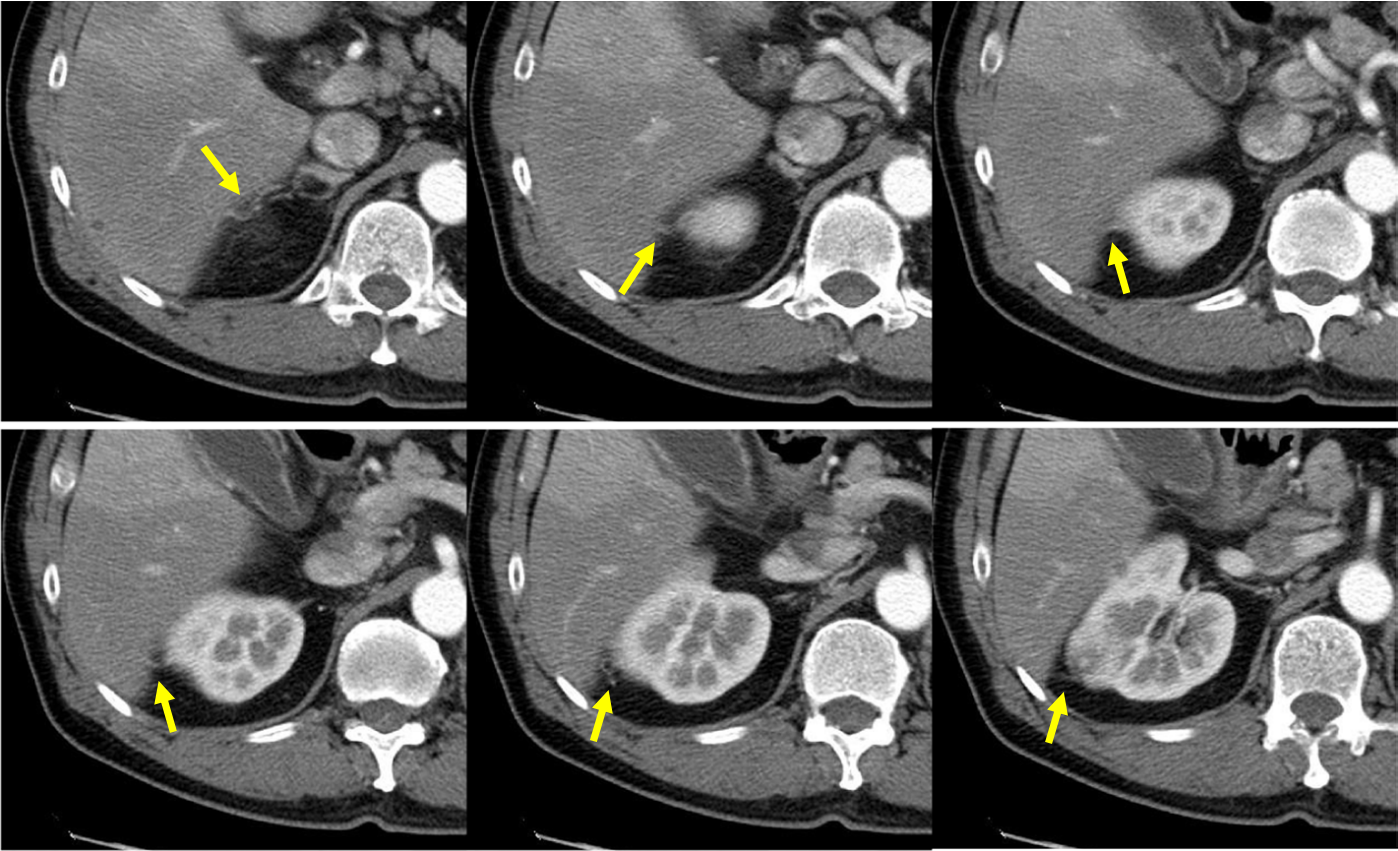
Representative images showing the appearance of CVs on CECT. We defined CVs as blood vessels of any diameter, with a definite connection between the tumor and the perirenal region. The yellow arrows indicate the CVs. CV, collateral vessel; CECT, contrast-enhanced computed tomography
